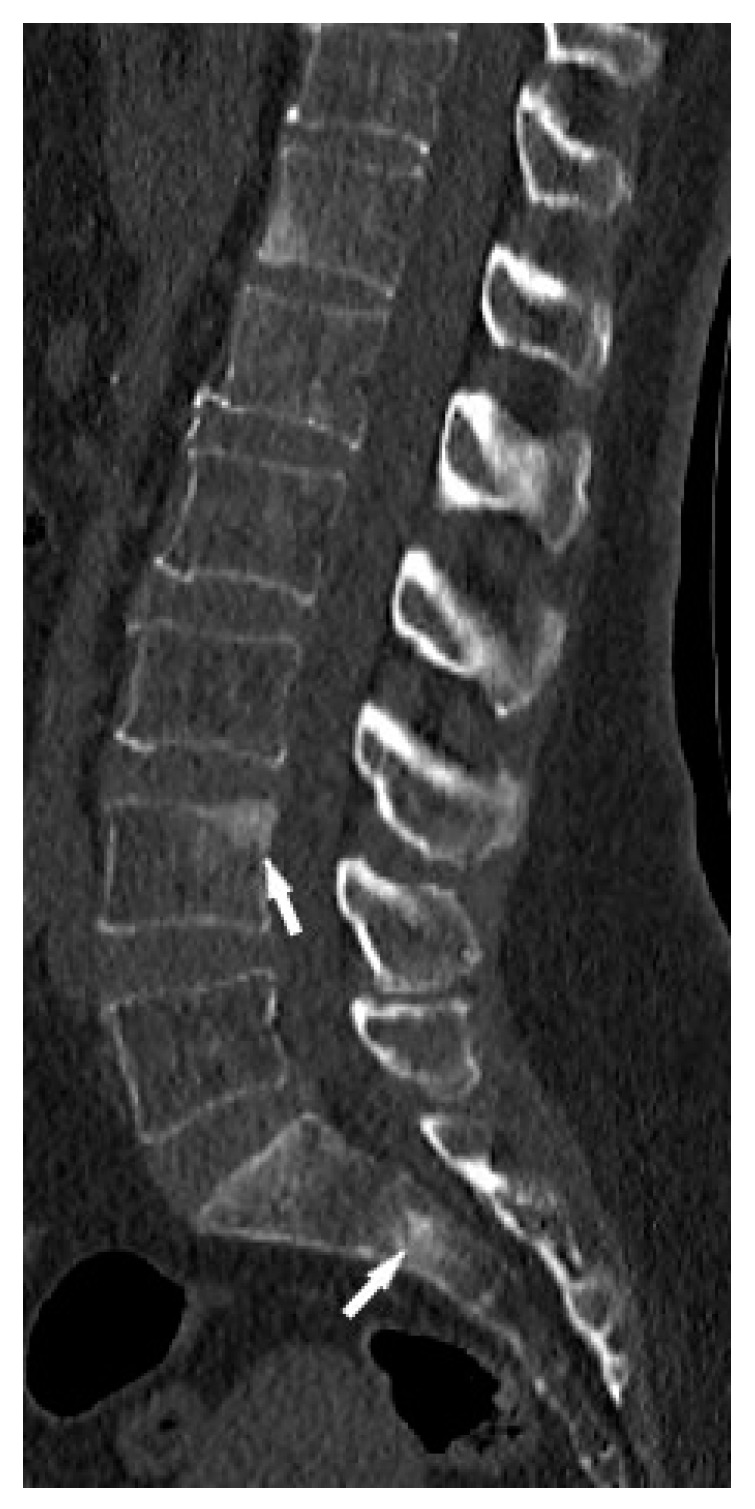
Lumbar spine CT, bone window. Osteosclerotic lesions (white arrows) in the L3 and S2 vertebral bodies.
